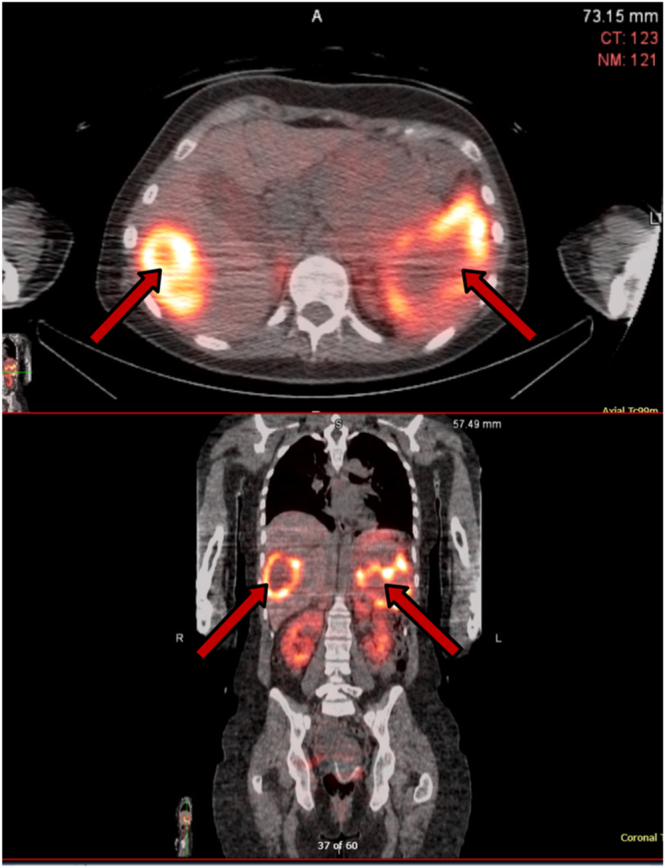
Case 2: octreotide scan with SPECT/CT images in axial (top) and coronal (bottom) views showing intense radiotracer uptake in a pancreatic mass and a liver metastasis (red arrows), consistent with Krenning 4 avidity.
